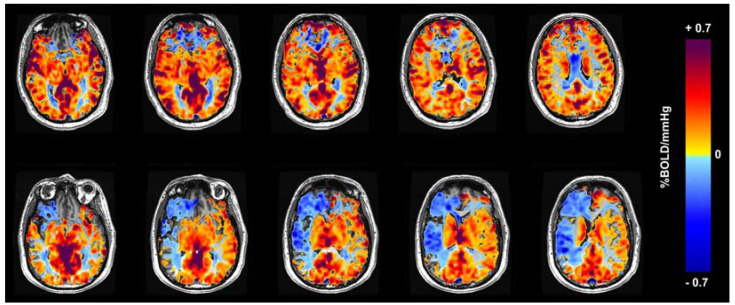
Colored CVR maps overlaid on the corresponding anatomic T1 weighted images (note: MRI images are displayed from the perspective of the feet looking up, so the right side of the brain is on the left side of the image). Positive CVR is yellow−red and negative CVR (steal physiology) is blue. Top row: normal healthy control. There is a susceptibility artifact from the skull base, which causes artifactually decreased CVR in the anterior inferior frontal lobes. Bottom row: patient with right−sided steno−occlusive disease and extensive steal physiology in the right middle cerebral artery (MCA) territory (blue which is on the left side of the images).
